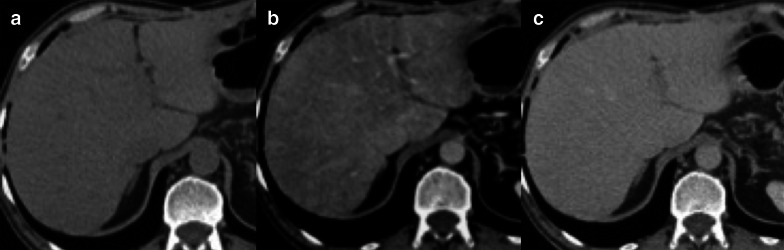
64-year-old man with history of left hemicolectomy for colon carcinoma and liver metastases treated with oxaliplatin. Contrast enhanced CT on precontrast (a), portal venous (b) and delayed (c) phases demonstrates a patchy liver enhancement with a mosaic appearance on portal venous phase, mainly in the periphery of the right lobe, with normal parenchymal enhancement on subsequent delayed phase. These findings are consistent with oxaliplatin-induced sinusoidal obstruction syndrome in its diffuse form
